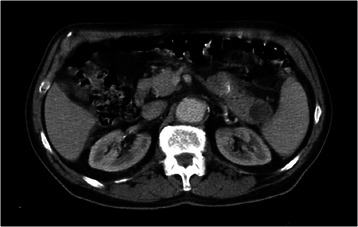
Abdominal CT with contrast: caudal pancreatic pseudocyst.
